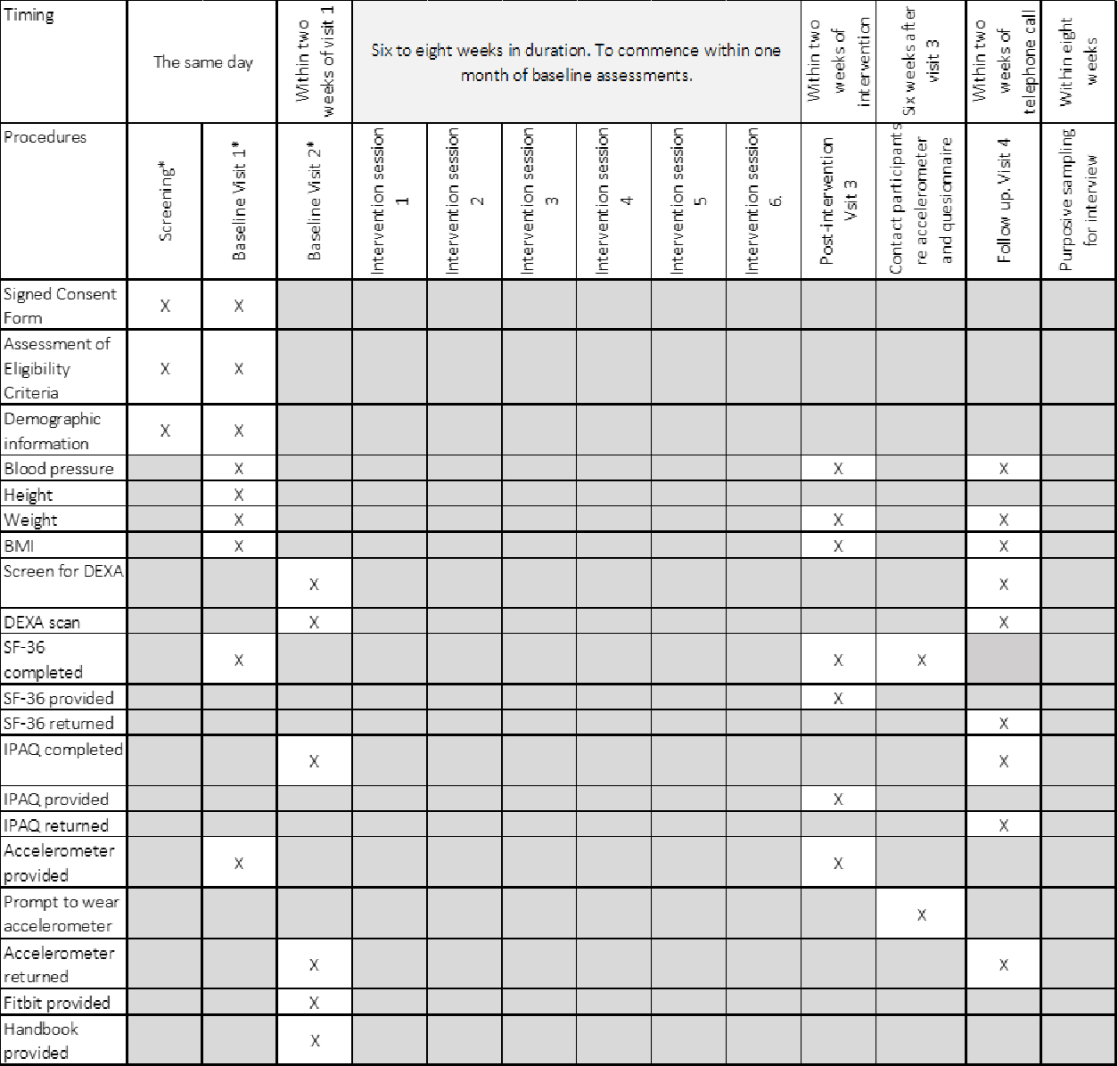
Schedule of events. BMI, body mass index; DEXA, dual energy X-ray absorptiometry; IPAQ, International Physical Activity Questionnaire; SF, short form.
